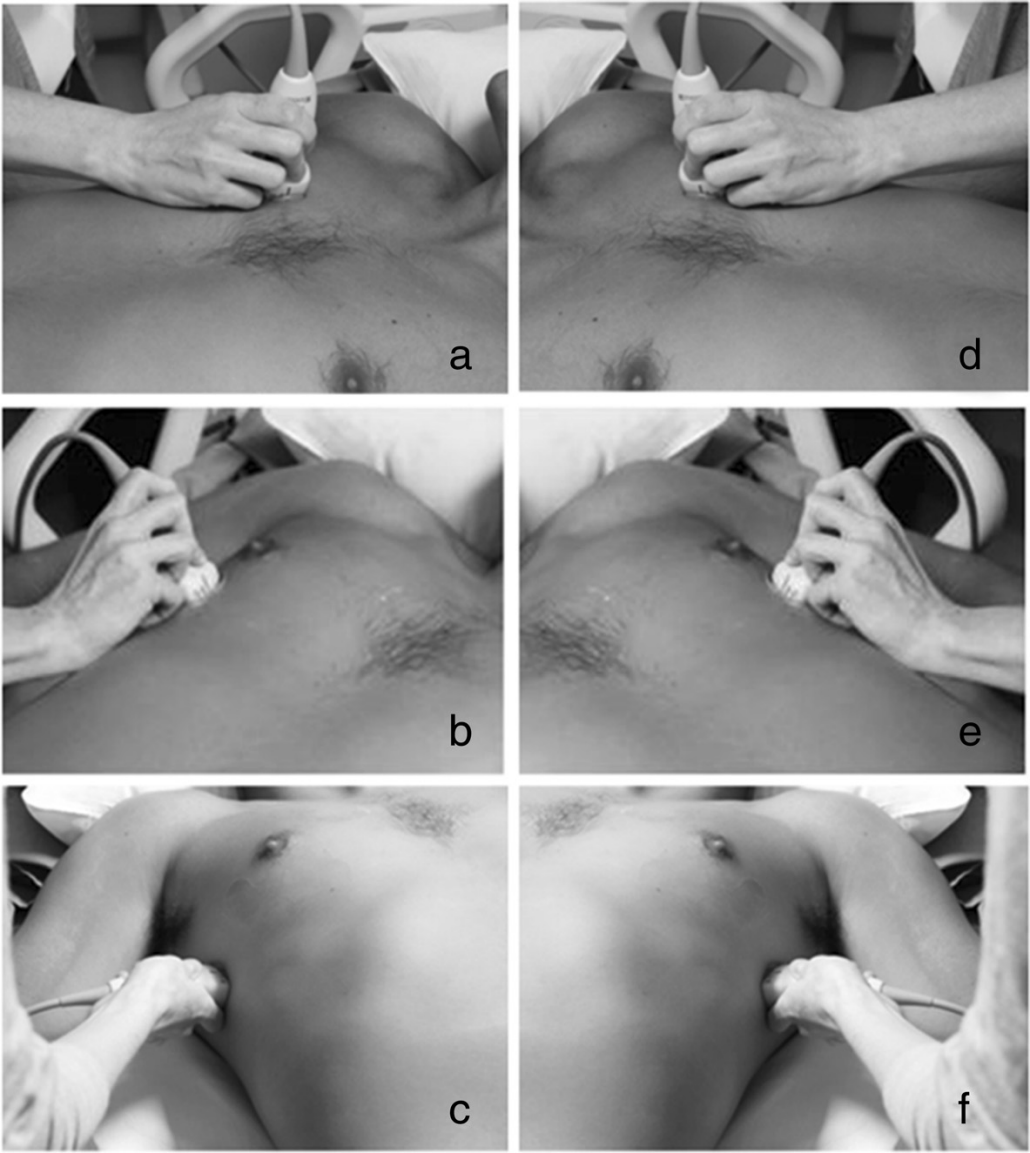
Placement of the ultrasound probe at six locations on the chest. a zone 1 is 2 cm below the anterior mid-clavicular line on the right side of the chest; b zone 2 is 4 cm inferior and 4 cm lateral to zone 1; c zone 3 is 2 cm inferior to zone 2 along the mid-axillary line. d–f The identical positions on the left side of the chest
